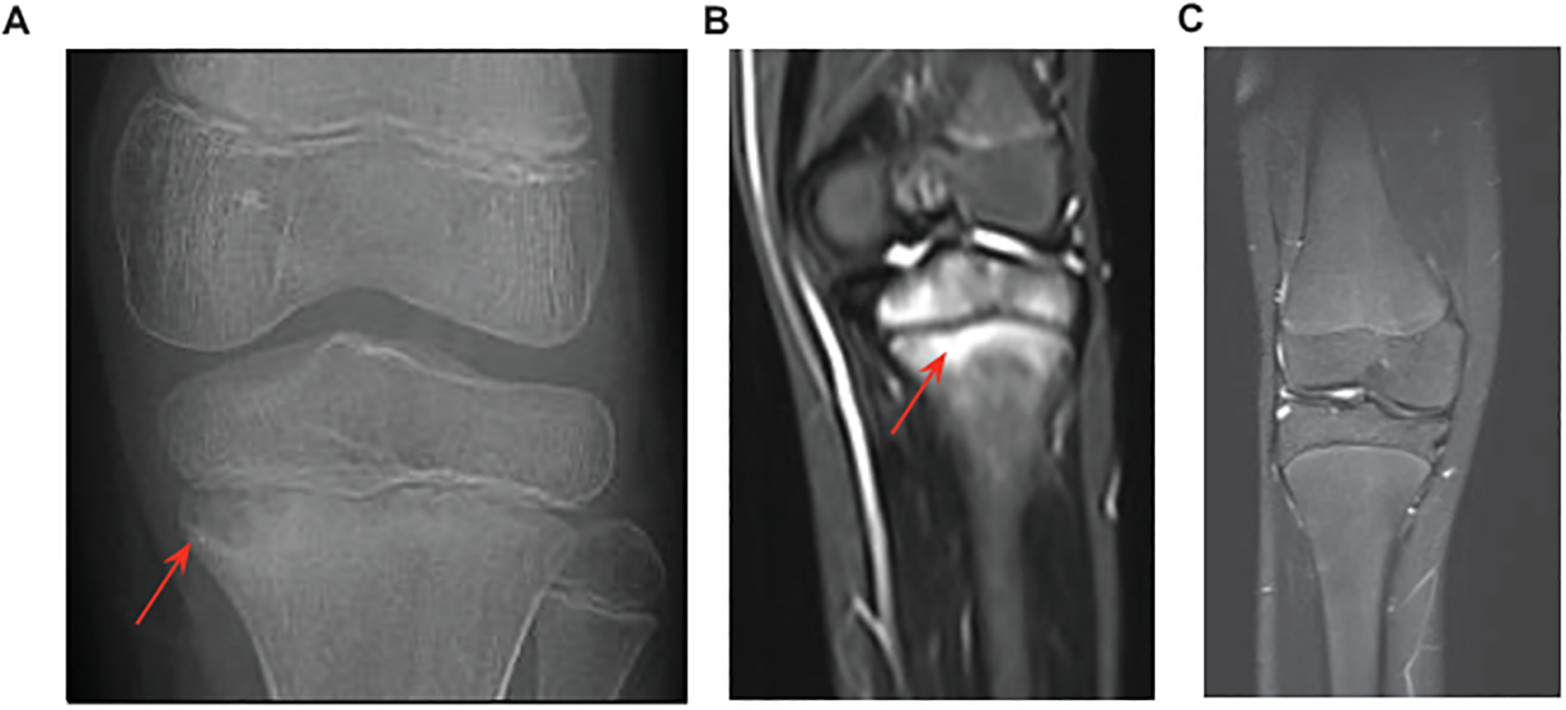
X-ray and MRI knee images from the affected proband. (A) X-ray showing osteomyelitis and a lesion characteristic of CRMO (red arrow) in the left proximal tibia. (B). MRI of the same knee showing inflammation and bone destruction (red arrow). Similar lesions were found in the clavicle, hip, femur, tibia, foot and toes (not shown). (C) MRI of a healthy knee for comparison.
